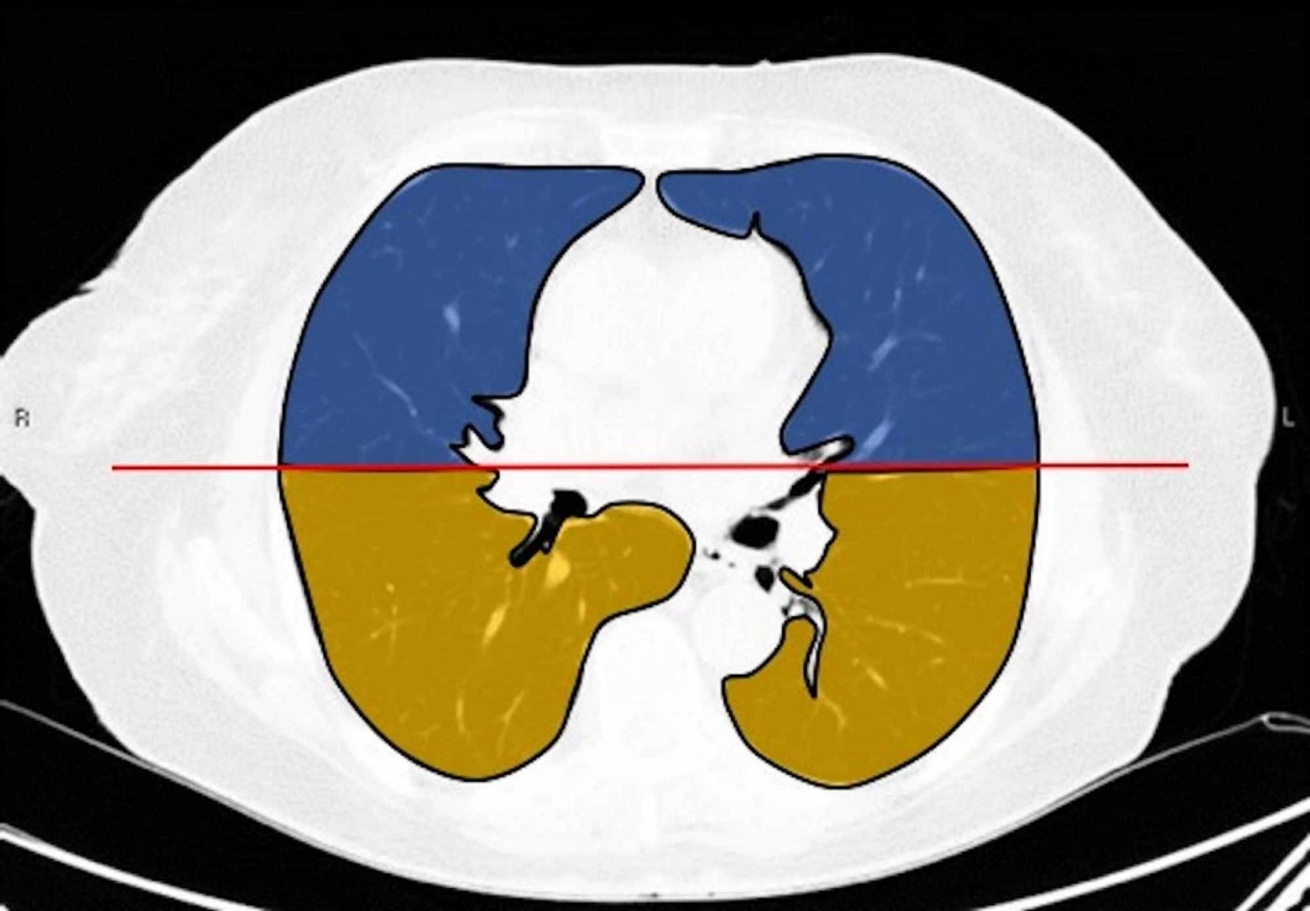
Division of lung fields in the axial plane Dividing lung fields equally into two axial planes on CT: blue represents the anterior half; brown represents the posterior half CT: computed tomography
